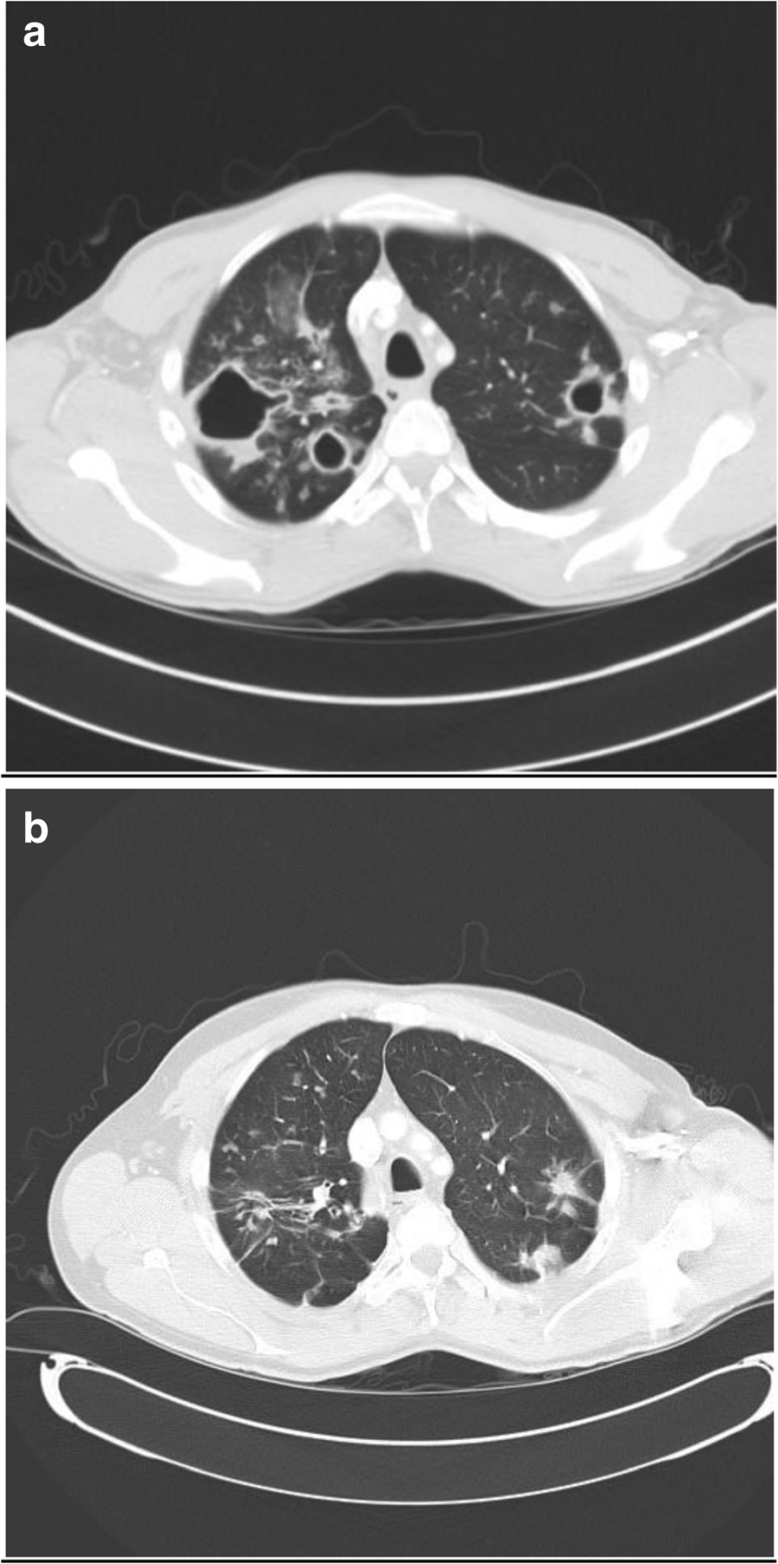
CT imaging showing cavitating disease initially, which developed into nodular disease
